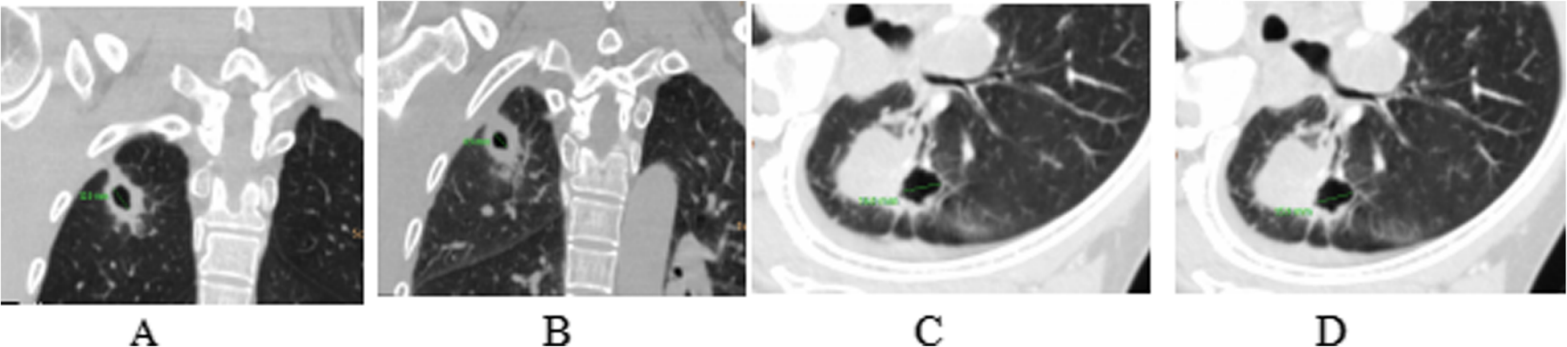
Typical CT findings. a and b were the same patient, male, 62 years old, with recurrent cough and sputum for more than 1 year, which was confirmed to be pulmonary tuberculosis by pathology. a: The maximum diameter of the cavity after deep inspiration was 12.0 mm. b: The maximum diameter of the cavity after deep exhalation was 8.9 mm. c and d were another patient, male, 67 years old, cough for more than 2 months, confirmed by pathology as lung cancer. c: The maximum diameter of the cavity after deep inspiration was 16.0 mm. d: The maximum diameter of the cavity after deep exhalation was 15.9 mm
